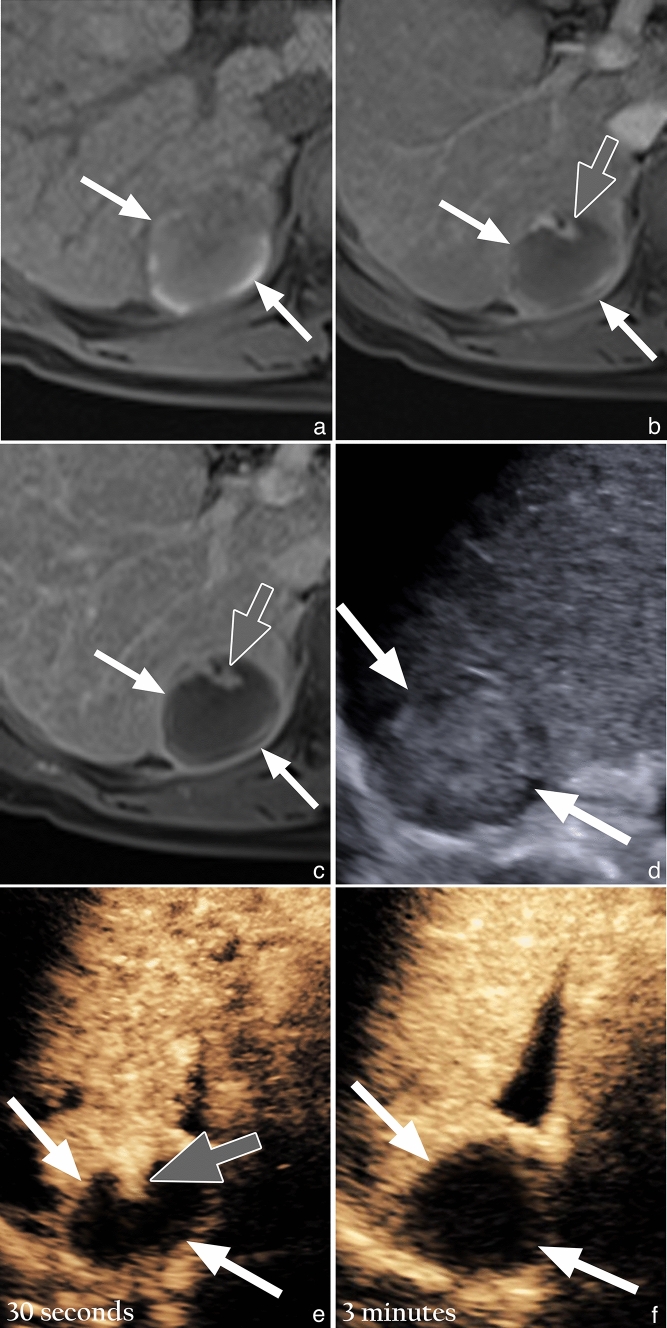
Resolution of the discordant appearance of intrazonal recurrence on MRI and CEUS, after RFA in a 70-year-old man with HBV cirrhosis. a) Unenhanced T1 weighted fat suppressed (T1FS) image shows a mildly hypointense treatment site (white arrows) with curvilinear arrows of hyperintensity at the periphery of the treatment site. b) Arterial phase T1FS subtracted image shows a mildly hyperenhancing nodular focus (gray arrow), within the anterior aspect of the treatment site (white arrows). c) Delayed phase T1FS subtracted image shows persistent enhancement (gray arrow) of the mildly arterially hyperenhancing focus, with no convincing evidence of washout. d) Gray-scale US image shows a mildly hyperechoic treatment site (white arrows). e) CEUS image obtained 30 seconds after microbubble contrast injection shows a nodular area of hyperenhancement (gray arrow) in the anterior aspect of an otherwise avascular treatment site (white arrows), corresponding to the mildly arterially hyperenhancing focus seen on the MRI. f) CEUS image obtained at 3 minutes shows washout of the arterially hyperenhancing nodular focus, in keeping with washout, leaving a completely avascular treatment site (white arrows)
